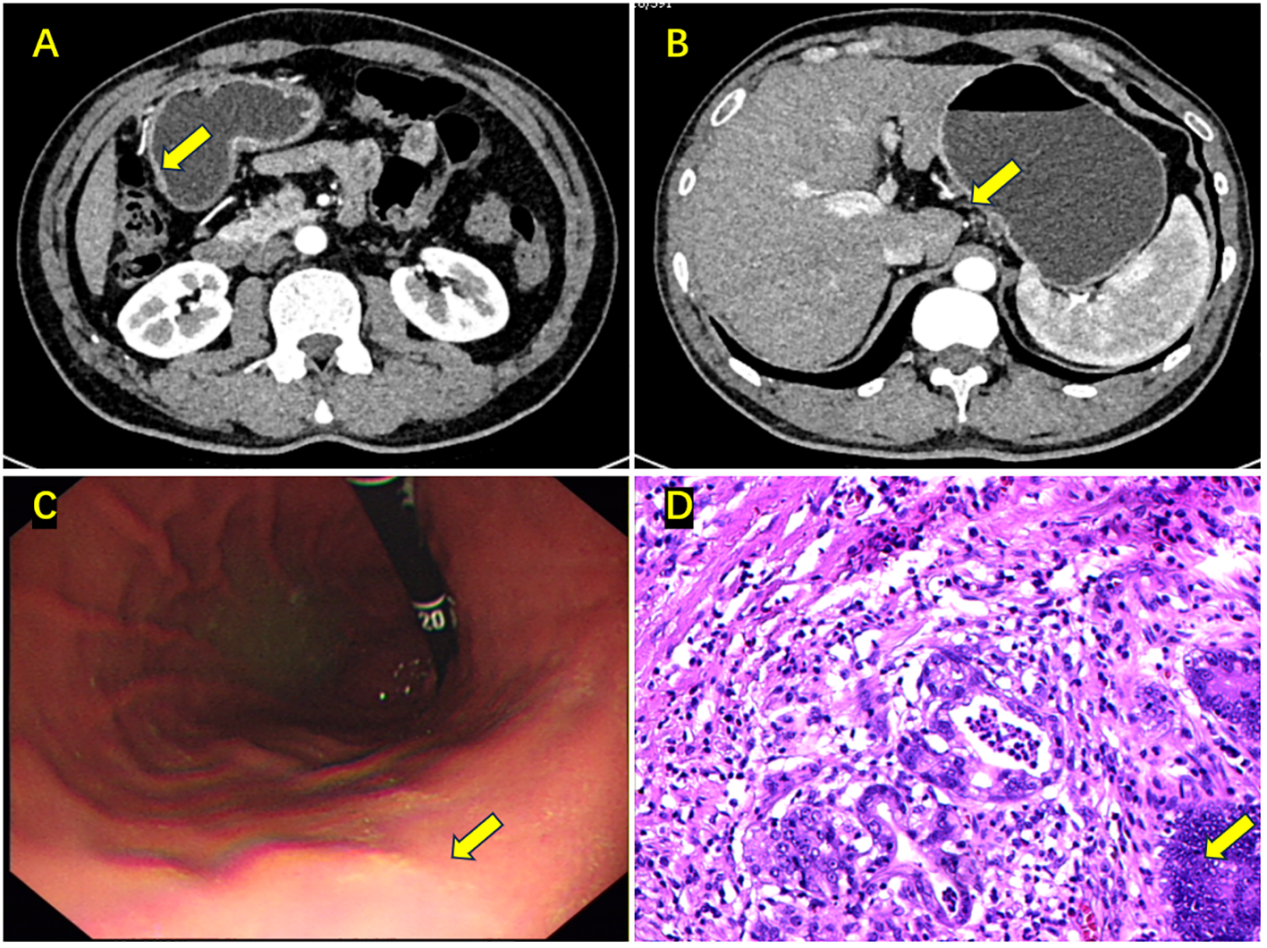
Enhanced abdominal CT, gastroscopy, and postoperative pathology results after neoadjuvant chemotherapy. (A) The tumor in the gastric antrum has almost disappeared, with a rough gastric wall (the yellow arrow); (B) The enlarged lymph node on the lesser curvature side has disappeared (the yellow arrow); (C) The mass in the gastric antrum has disappeared, with mucosal congestion and edema (the yellow arrow); (D) Postoperative pathology: ypT1aN0M0, tumor nests located in the lamina propria (the yellow arrow).
